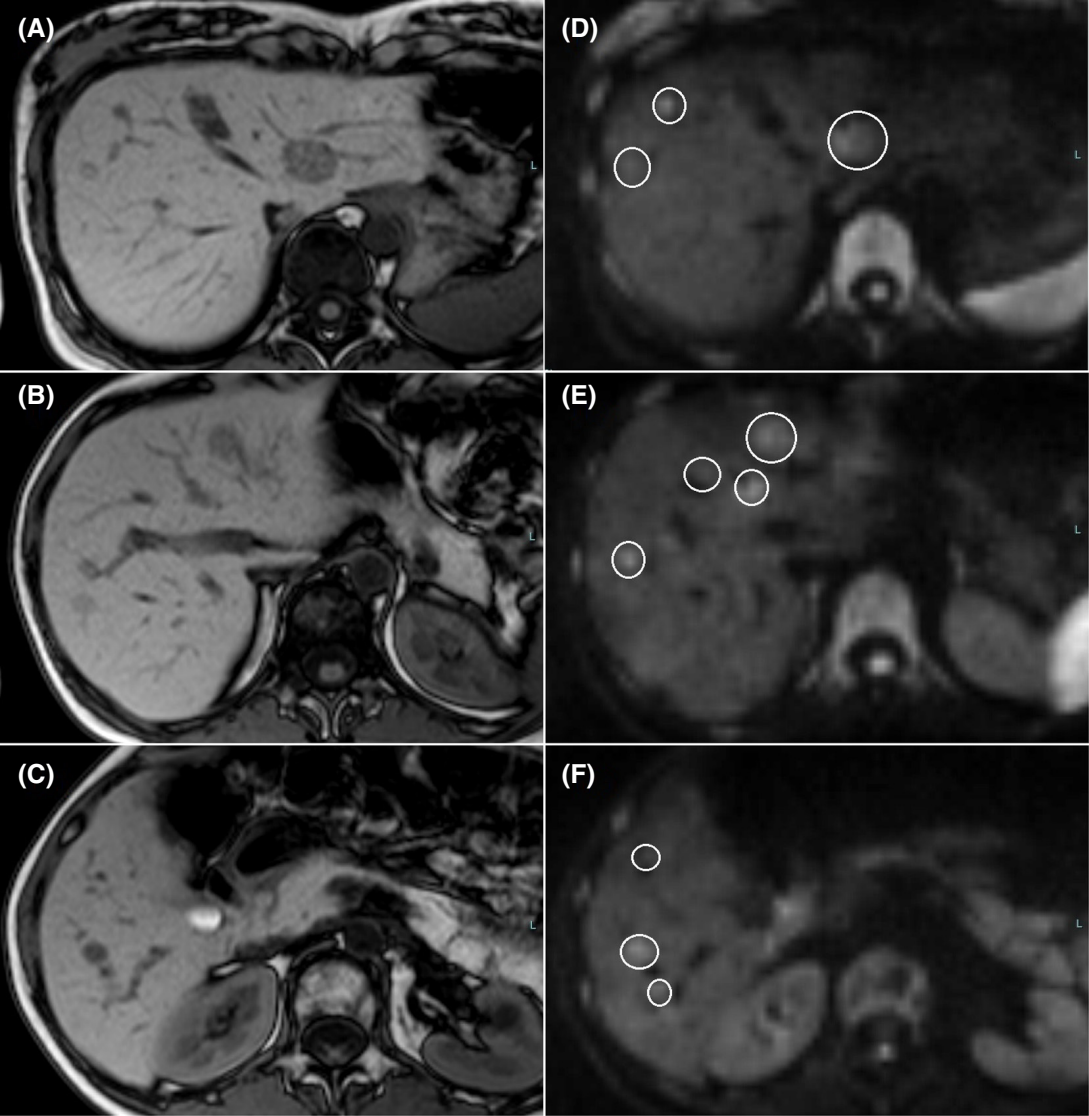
Axial T1‐weighted MRI images (A–C) and diffusion‐weighted images (D–F) show at least 10 lesions (white circles) at 30 weeks of gestation. HCC, hepatocellular carcinoma; MRI, magnetic resonance imaging.
